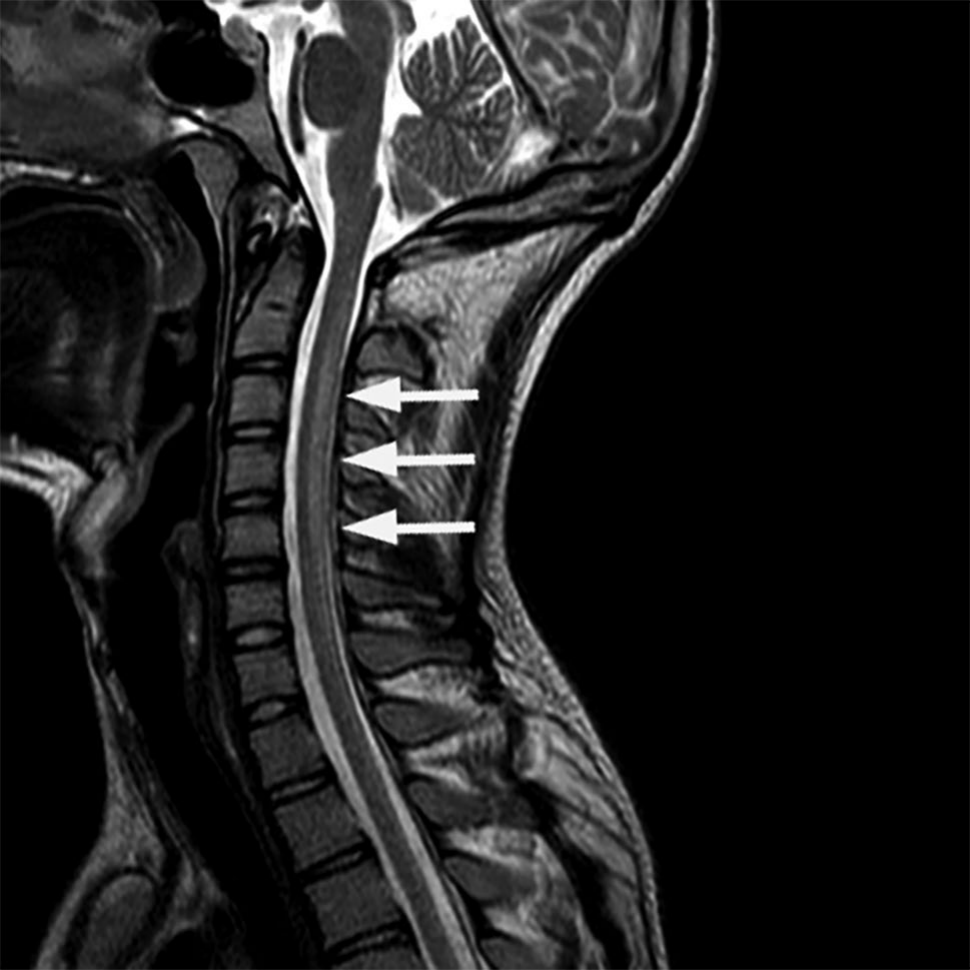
T2-weighted MRI; sagittal view showing increased signal in the dorsal columns from C2 to C7 (white arrows)
